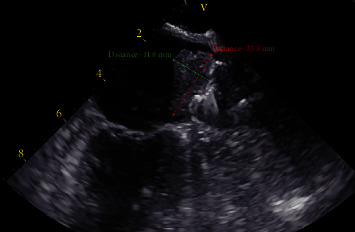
Transesophageal echocardiogram measuring a large thrombus within the left atrium atop an implanted WATCHMAN FLX™ device.
